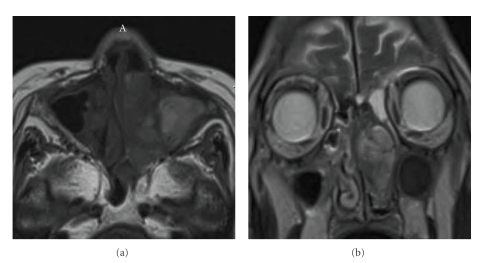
Horizontal (a) and axial (b) magnetic resonance imaging (MRI) showed a nasal tumor in the left nasal cavity with a hypointense signal on T1-weighted images, and a moderately hyperintense signals on T2-weighted images. This signal was different from that of the left maxillary sinus lesions.
